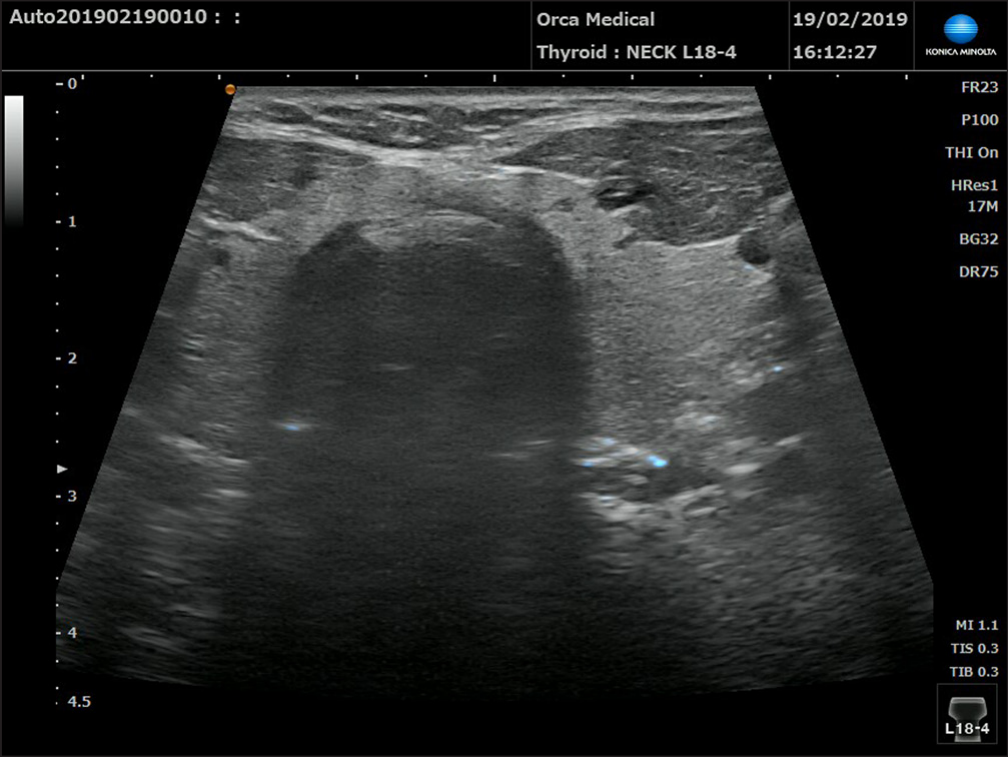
Position of the needle on ultrasound view. Blue dot on the left side representing the tip of the EMG needle, going in the Longus Colli muscle through the thyroid gland (SNV mode). Small cysts, benign in appearance, can be seen in the thyroid.
